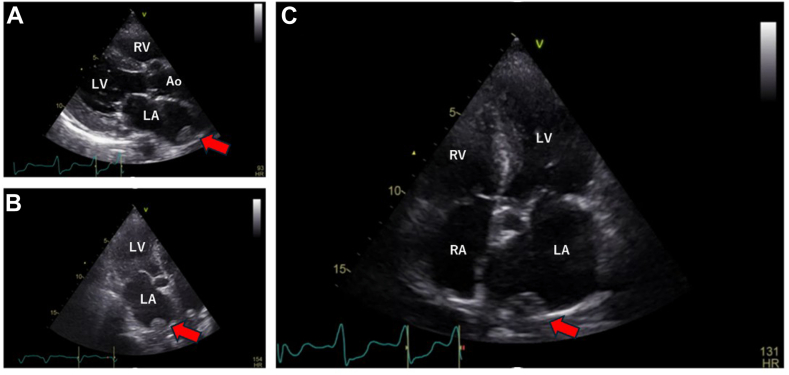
Two-dimensional TTE, parasternal long-axis (A), apical 3-chamber (B), and apical 4-chamber (C) systolic views, demonstrates the dilated LV and LA and a large, nonmobile, hyperechoic mass *(arrows)* protruding into the posterior wall of the LA from the orifice of the RLPV. *Ao*, Aorta; *LV*, left ventricle; *RA*, right atrium; *RV*, right ventricle.
